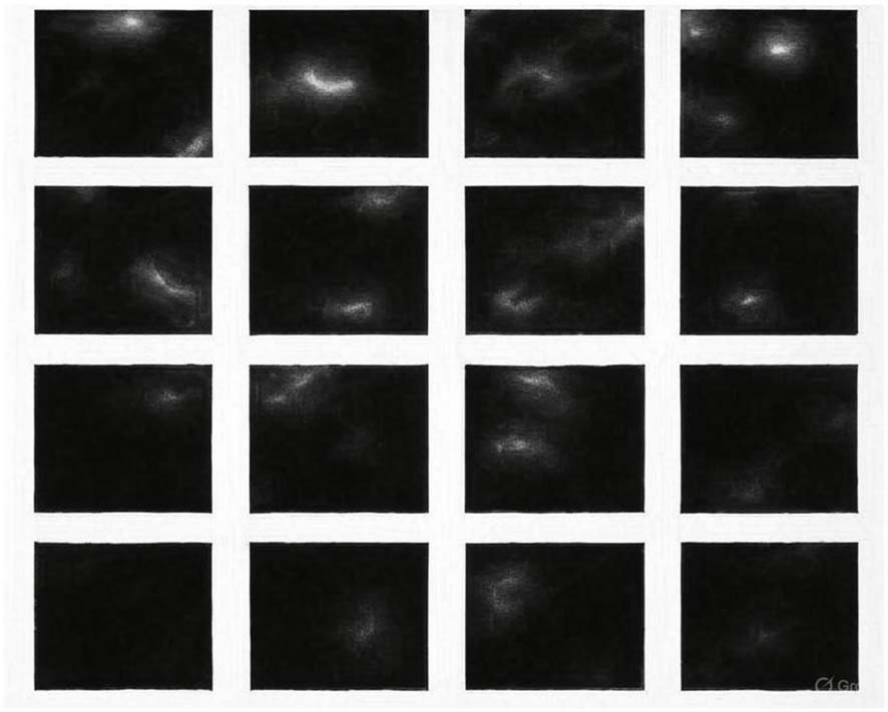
MRI Generated by the POP-GAN model.
